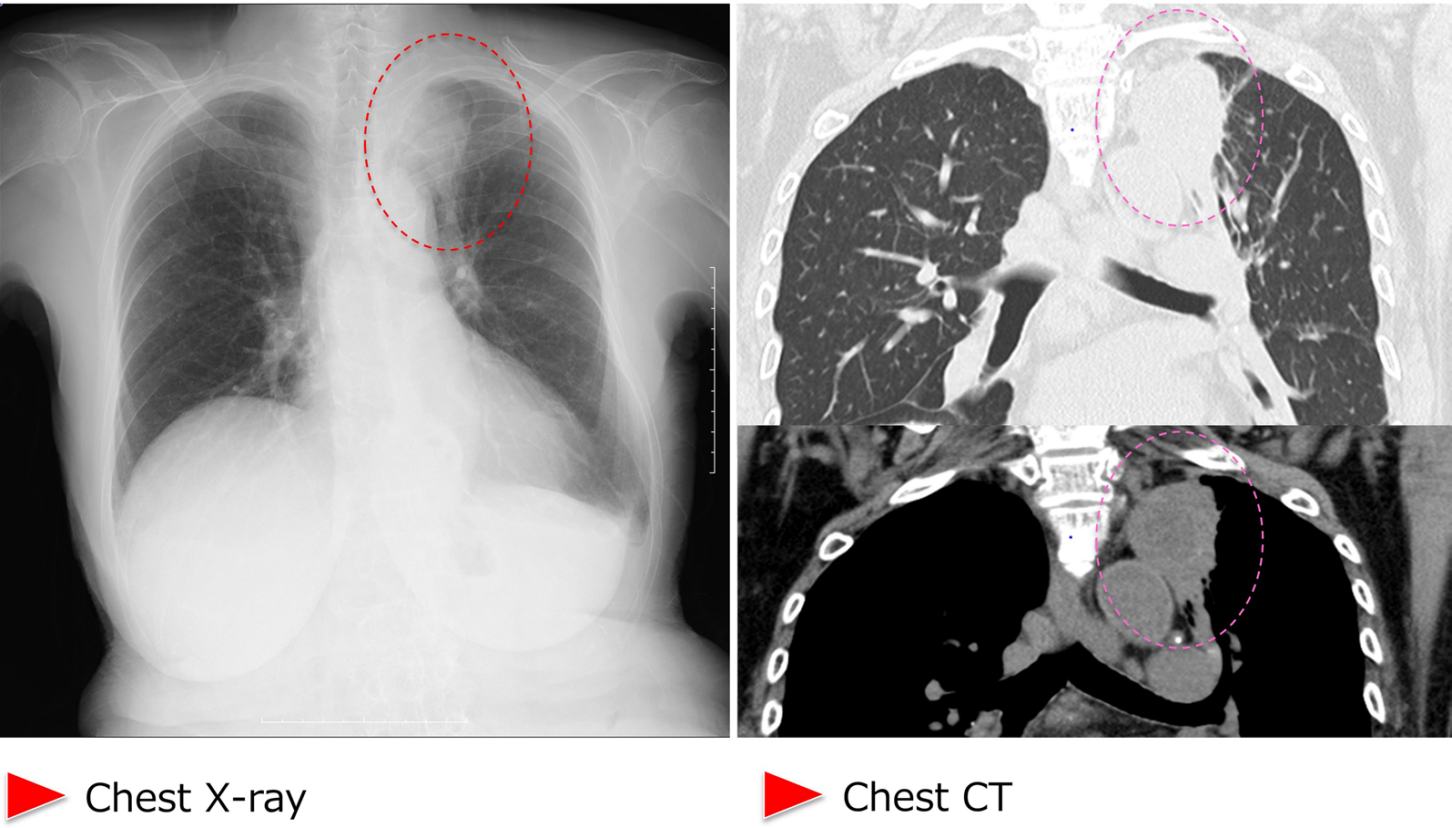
Chest X-ray and chest CT imaging of lungs with pulmonary aspergillosis. Images show a 43 mm nodular shadow in the upper lobe of the left lung. The dotted circle indicates the nodular shadow
